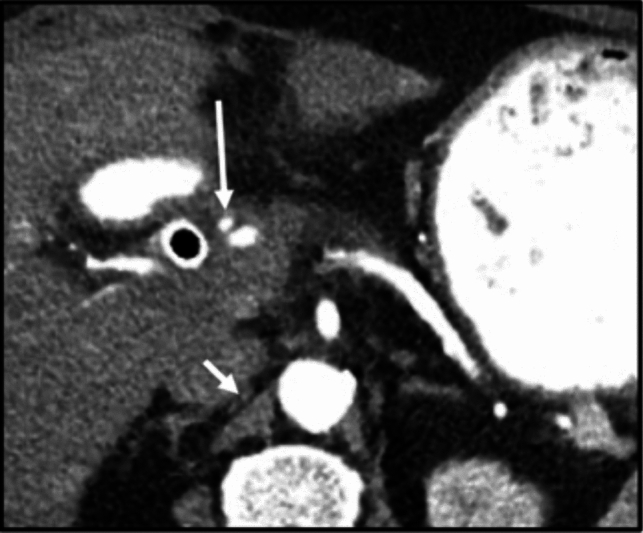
Axial CT section with arterial phase contrast. Ill-defined mass in the anterior pancreatic head, which encases the gastroduodenal artery (long arrow). Disease infiltrates close to the right celiac ganglion (short arrow)
